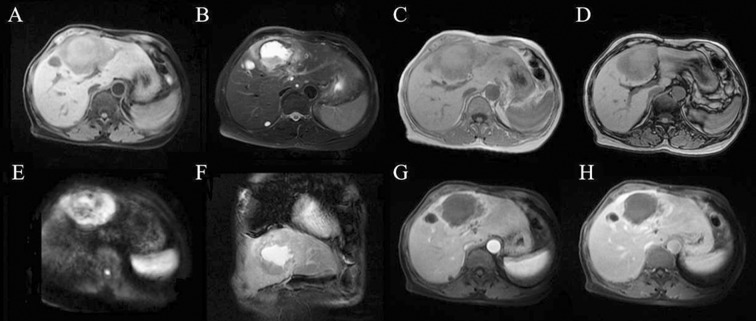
The different sequences of MRI showing the hepatic abscess in the area of the liver metastasis. T1WI (Fig.1 A), axial T2WI (Fig.1 B), coronal T2WI (Fig.1 F), dual echo T1WI (Fig.1 C, D), DWI (Fig.1 E), and LAVA (Fig.1 G, H) were performed.
